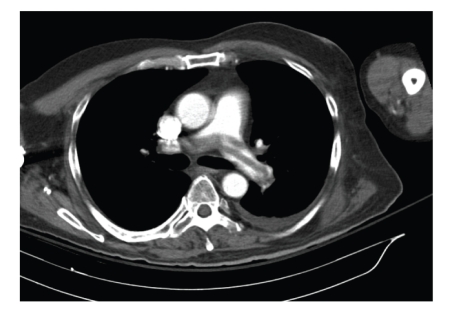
CT pulmonary angiogram showing a large saddle embolus.
